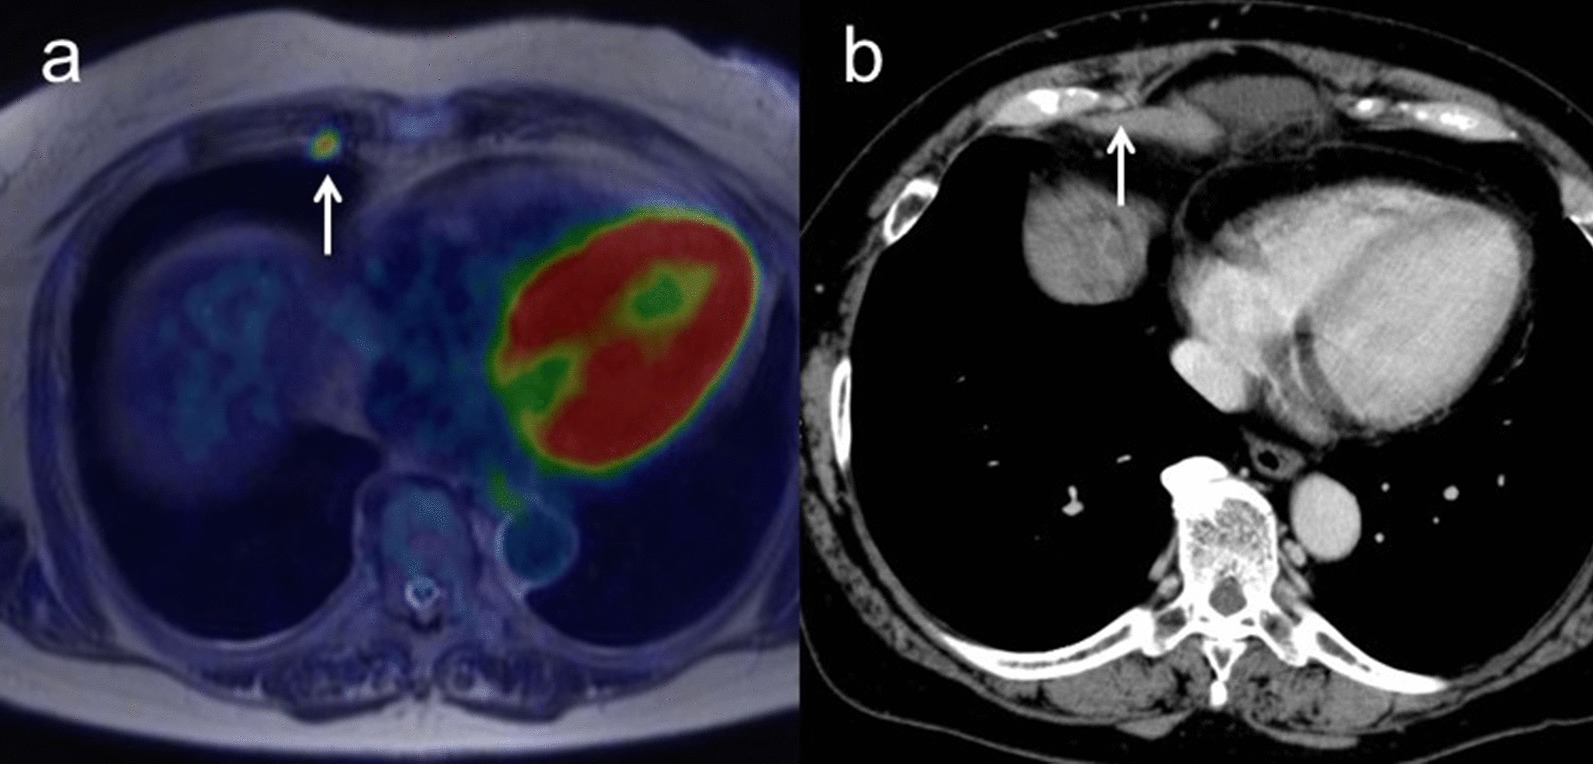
a A 66-year-old woman with pathologically confirmed ovarian cancer. Axial T2-weighted PET/MR image shows a right parasternal lymph node with FDG uptake (arrow). b Contrast-enhanced CT shows a right parasternal lymph node less than 1 cm in short-axis diameter with slightly enhancement (arrow). After NAC, this lymph node decreased in size and SUV, suggesting that this node as a sign of malignancy (M1)
